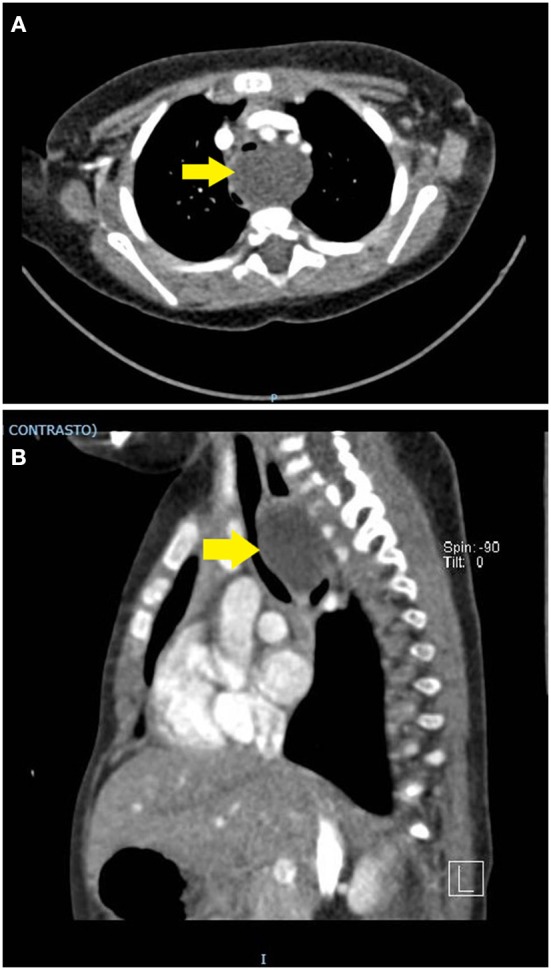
(A,B) Contrast-enhanced chest CT scan. A thin-walled round mass (antero-posterior to lateral diameter, size 30 × 25 × 40 mm) can be seen at the level of the first five dorsal vertebral somas (from D1 to D5). The mass (yellow arrow) occupies the upper and middle mediastinum, displacing the esophagus posteriorly and the trachea anteriorly. In dynamic acquisitions a marked reduction in the size of the trachea to the middle third is documented. The epiaortic vessels appear to be slightly displaced anteriorly without reduction in size.
